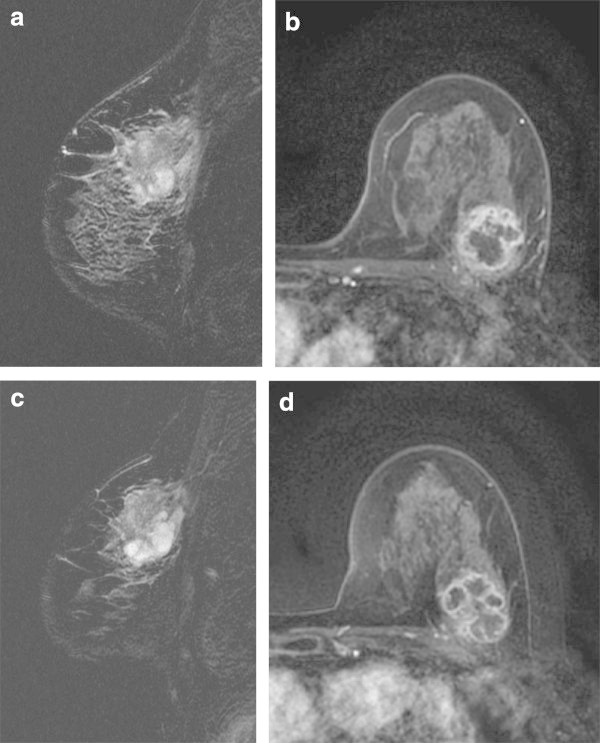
Triple-negative breast cancer of left breast in 69-year-old woman. Prior to NAC, fat-suppressed T2-weighted MR image shows a mass with an area of very high intratumoral intensity (a) and dynamic MR image obtained at 60 s after administration of gadolinium shows a peripherally-enhancing mass with a large area of intratumoral necrosis (b). After the completion of NAC, very high intratumoral intensity enlarges on fat-suppressed T2-weighted MR image (c) and there is an increase in tumor size on dynamic MR image obtained at 60 s (d).
